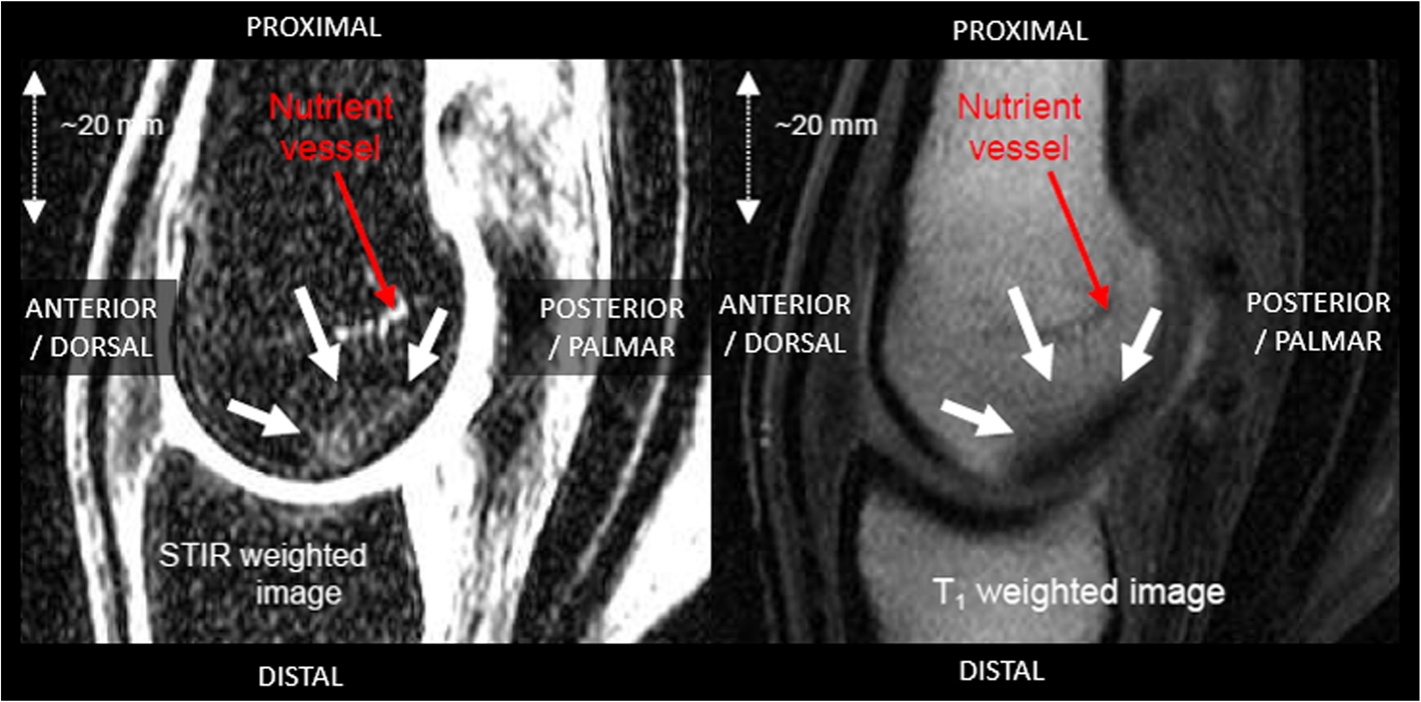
Mid-sagittal magnetic resonance images of the metacarpophalangeal joint. Arrows indicate the region of the BMOA
